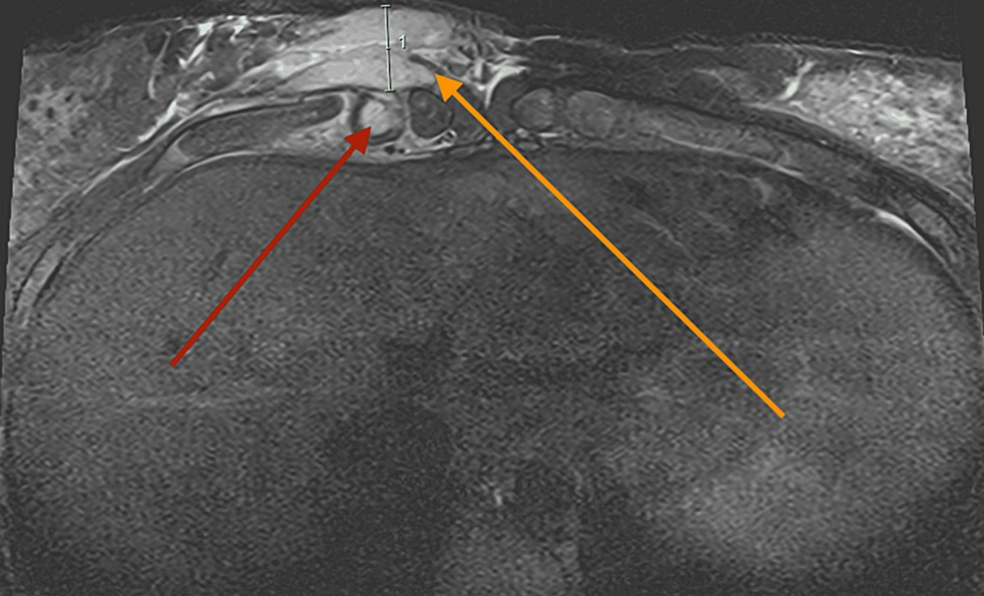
MRI chest wall (axial). Infective chondritis with associated subcutaneous inflammation. Red arrow: enhancing T2 hyperintense focus measuring 13x5mm, involving the mid costal cartilage on the right Orange arrow: associated subcutaneous inflammatory phlegmon/abscess (length: 19.61mm)
